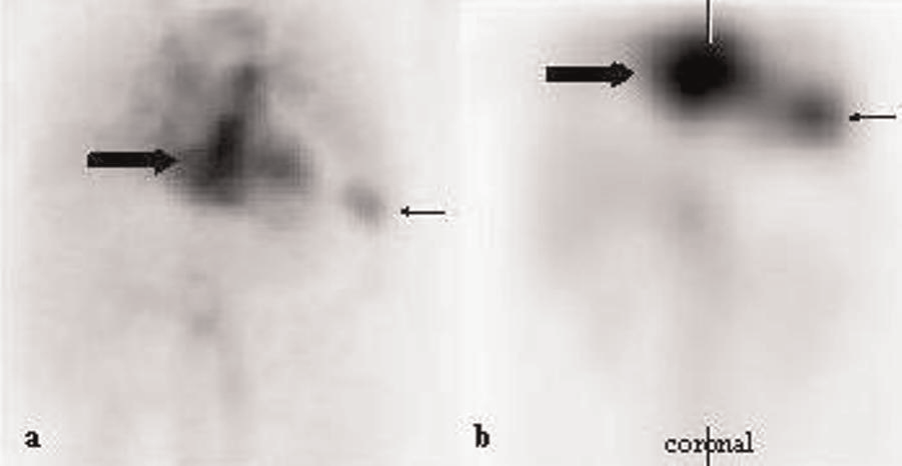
Tc-99m-DRBC scintigram. Heat-damaged and Tc-99 m-labeled autologous red blood cell scintigram shows uptake of the labeled cells in the left lobe of the liver (thick arrow) and in the vicinity of the removed spleen (thin arrow) in the planar scan (a) and the SPECT-images (b) corresponding to the masses seen in MRI and CT.
